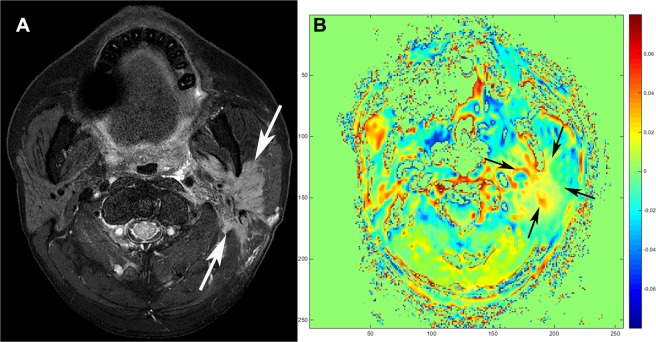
Squamous cell carcinoma of a 55-year-old male. (A) Axial T2-weighted imaging with fat suppression shows an irregular mass with infiltrative margin and heterogeneous signal intensities in the left parotid gland (arrows). (B) Tumor shows heterogeneous signal on amide proton transfer-weighted (APTw)-MRI (arrows). Note the markedly increased asymmetry value at the solid portion of the tumor. The averaged APTw-signal values from the two readers were as follows: Mean APTw-signal, 2.44%; maximum APTw-signal, 10.22%; median APTw-signal, −2.53%.
